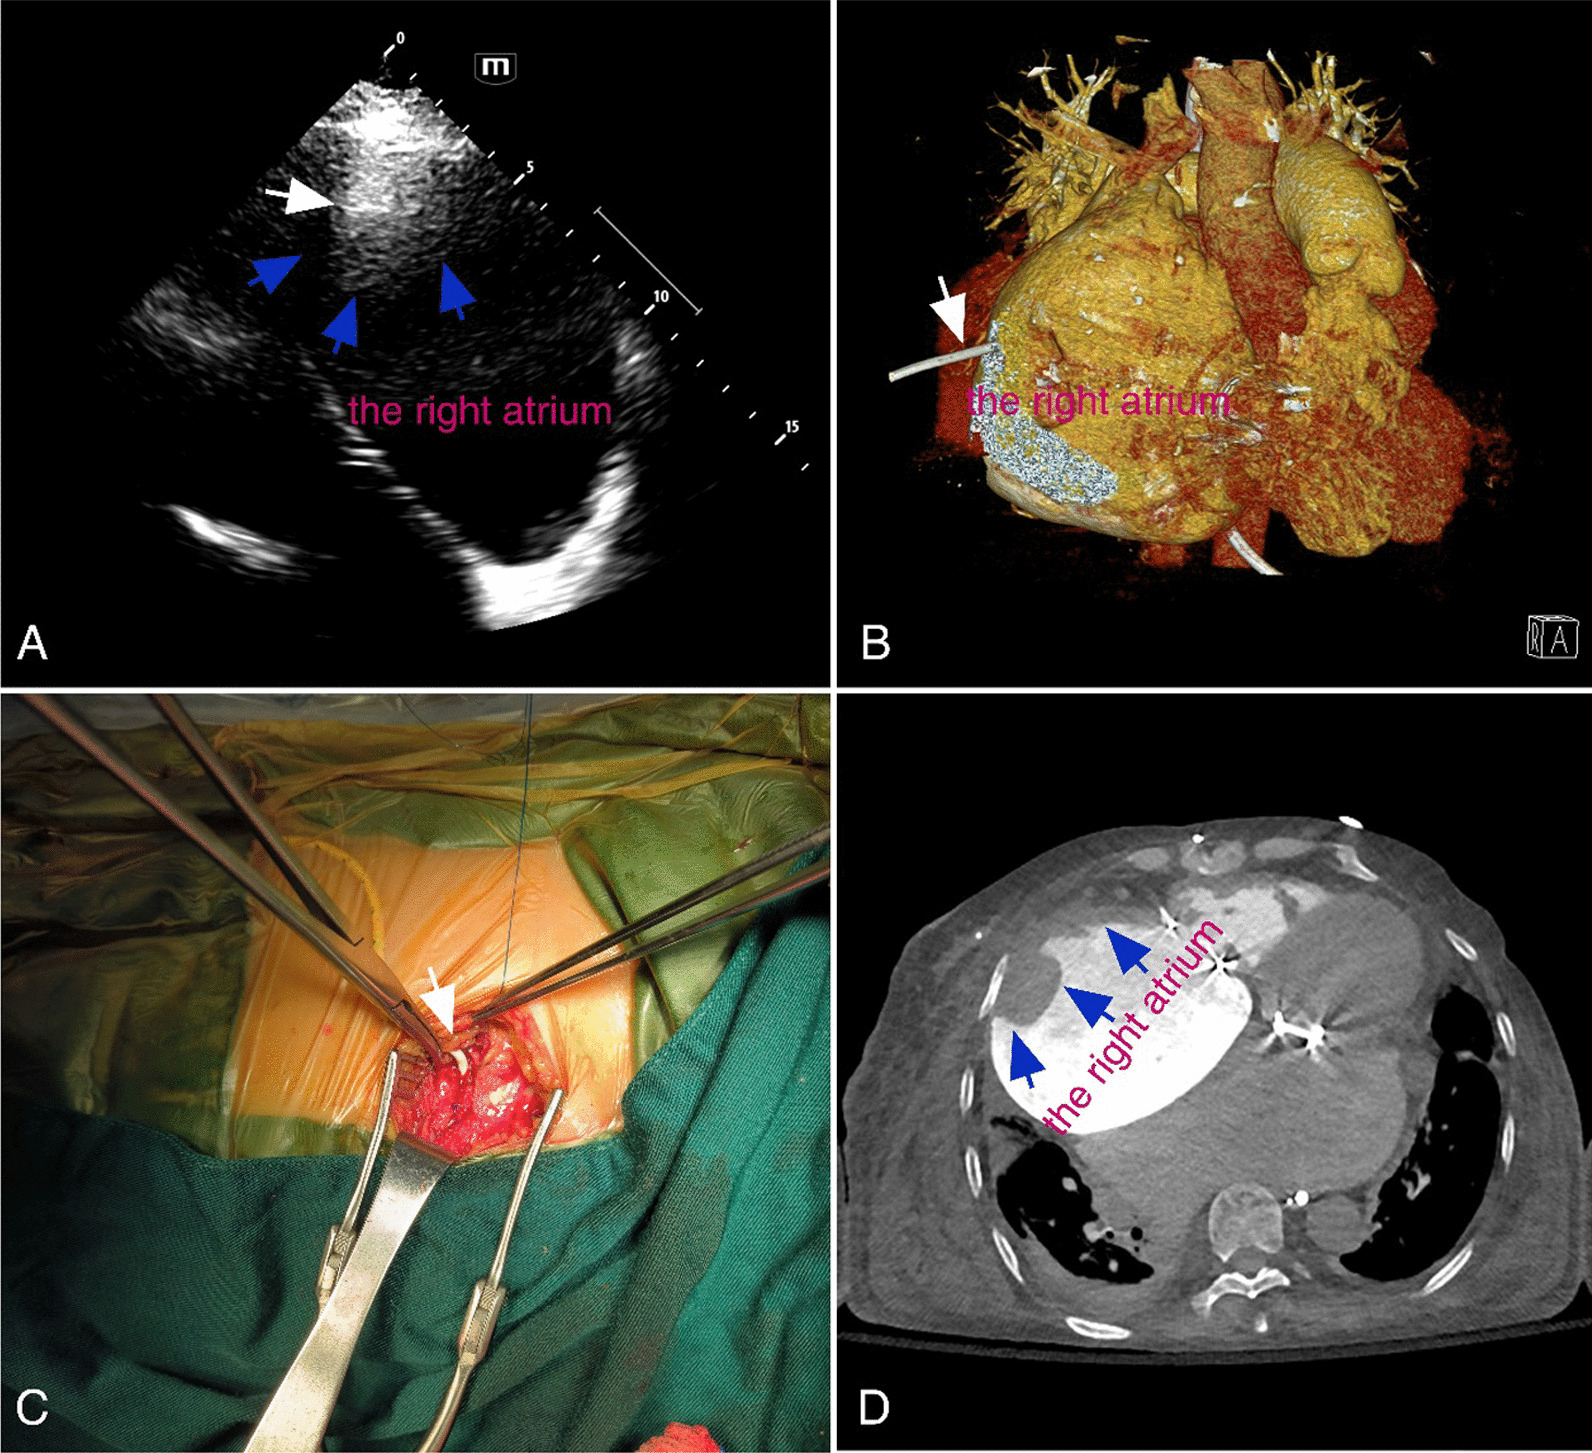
A Transthoracic echocardiogram demonstrating thrombus (blue arrows) in the right atrial wall with high-echo catheter tip stuck in (white arrow); B 3D CT reconstruction showing outer segment of the tube; C intraoperative finding of drainage tube inserted into the heart; D residual flake-like mural thrombus (blue arrows) in the right atrial wall after tube extraction
